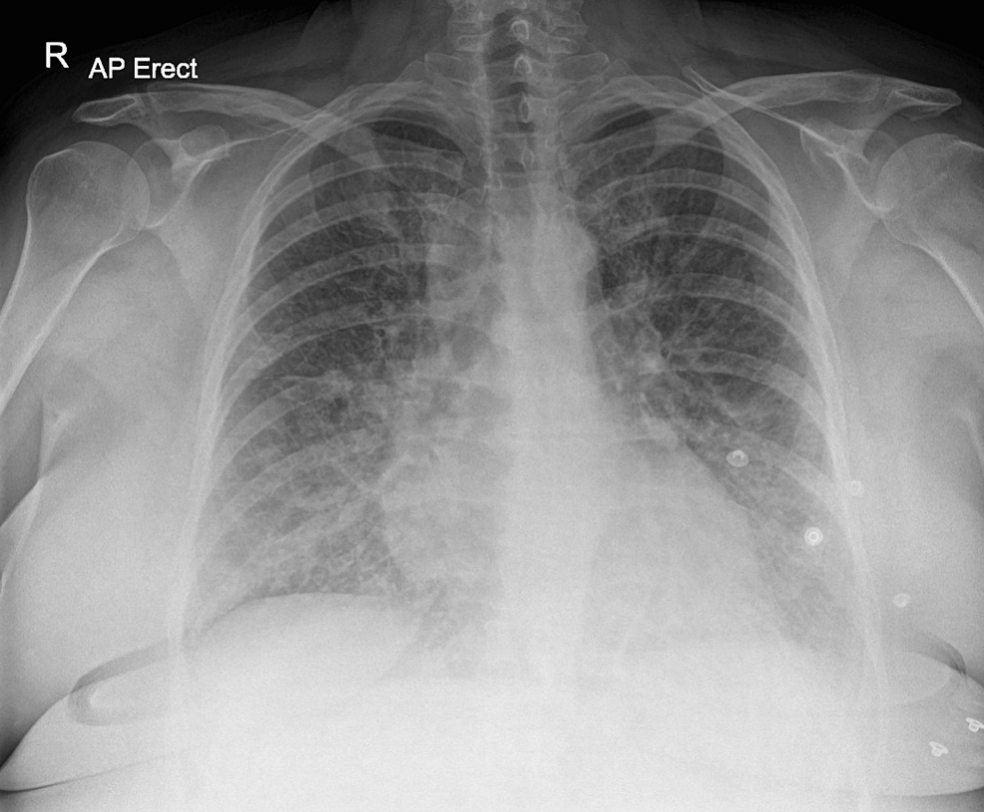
Chest radiograph demonstrating bilateral pulmonary infiltrates (case 2).
